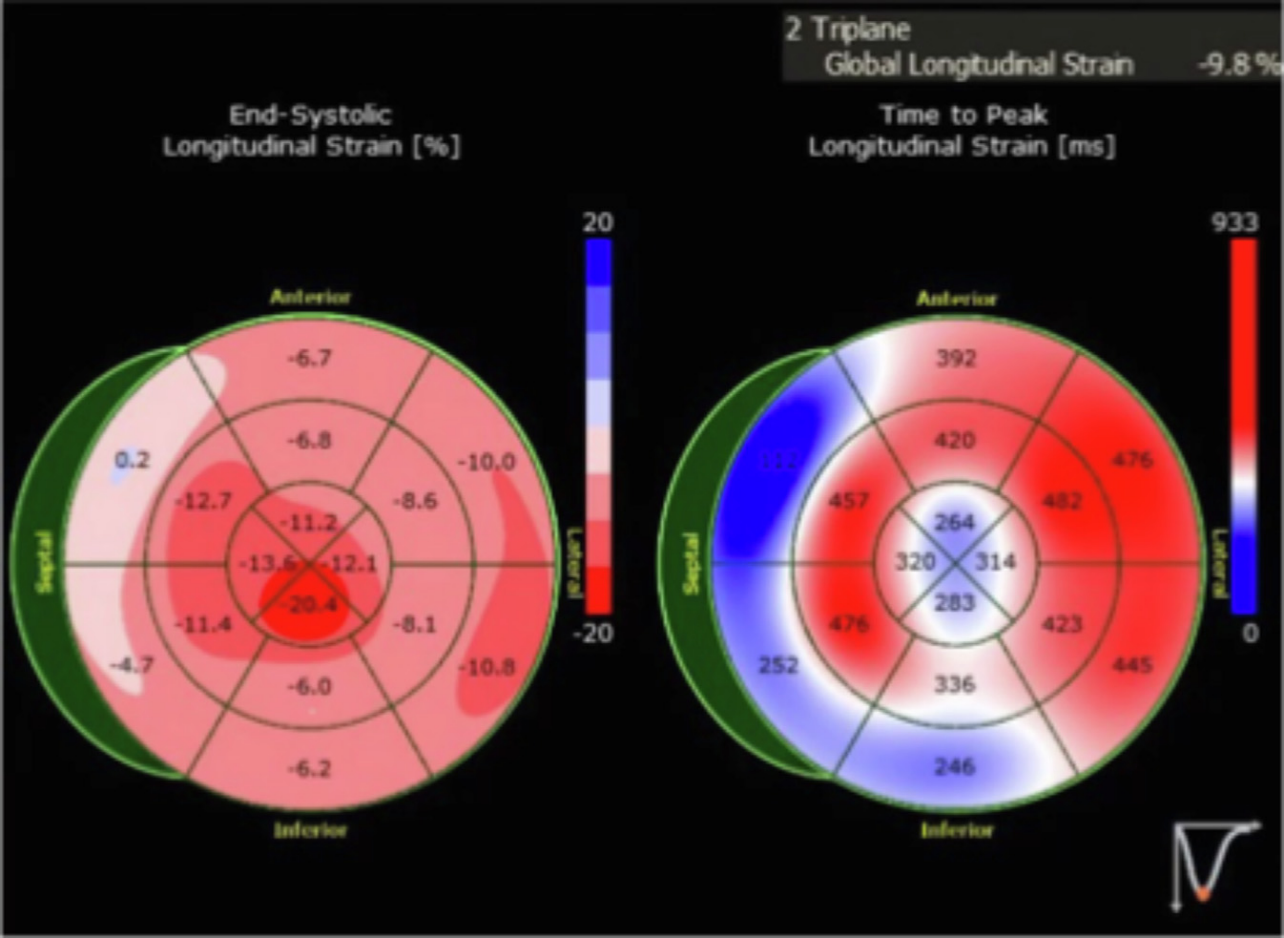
Patient 2 Had Reduced Global Longitudinal Strain on the Echocardiogram With Apical Sparing
